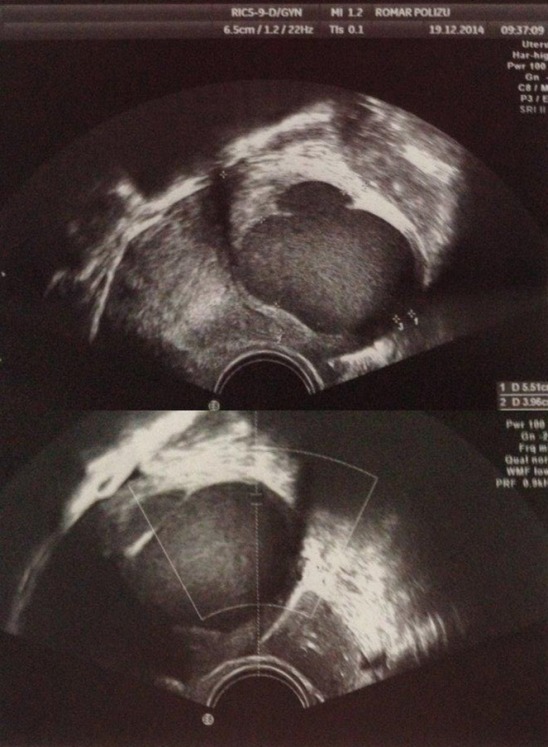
Preoperative color Doppler transvaginal ultrasound
